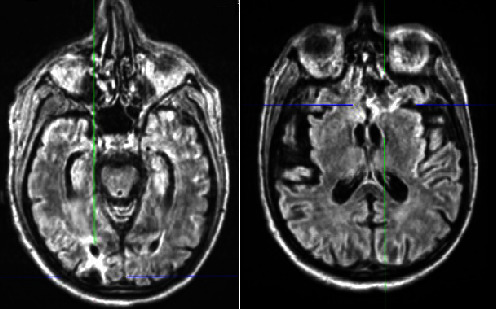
Cranial magnetic resonance imaging scan of the patient (axial, T1-weighted), which revealed left frontobasal and right occipital gliotic–malacic lesions with marginal hemosiderin deposits, suggesting posttraumatic or postischemic sequelae.
